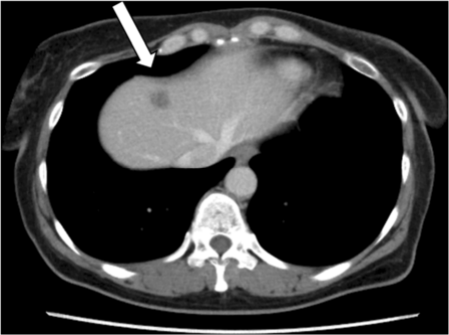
Axial slice on enhanced CT in the equilibrium phase. A low density area was detected in segment 4 of the liver (*arrow*)
